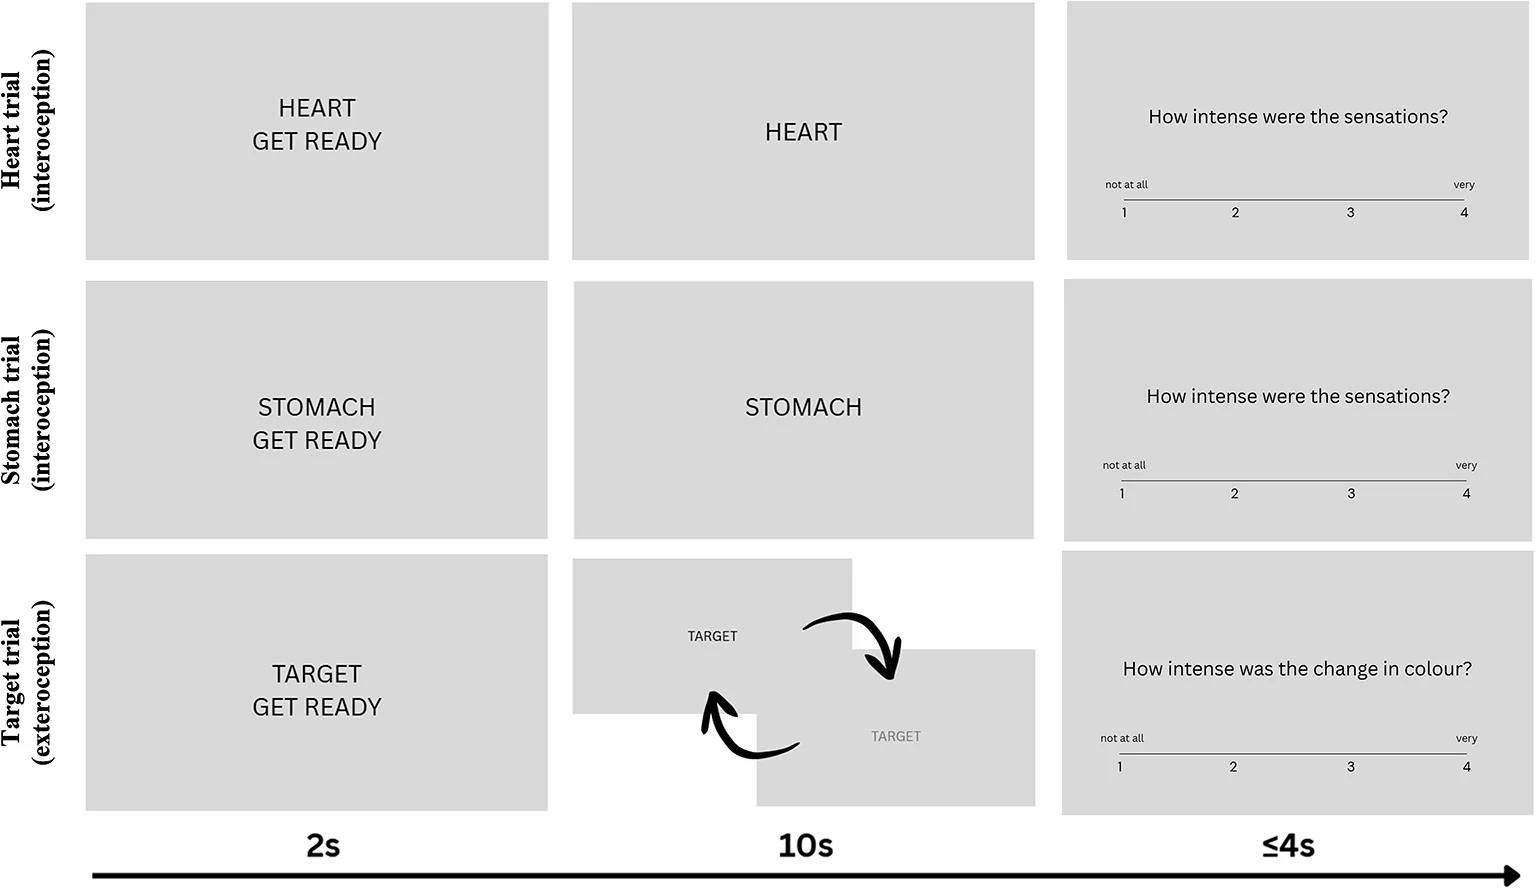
Procedure for functional magnetic resonance imaging interoception task. Stimuli are split according to trial type, including the interoceptive conditions of “heart” and “stomach” and the exteroceptive control condition of “target.” Stages of each trial are presented, including the “get ready” cue (2 s), the focus period (10 s), and the intensity rating period (up to 4 s).
